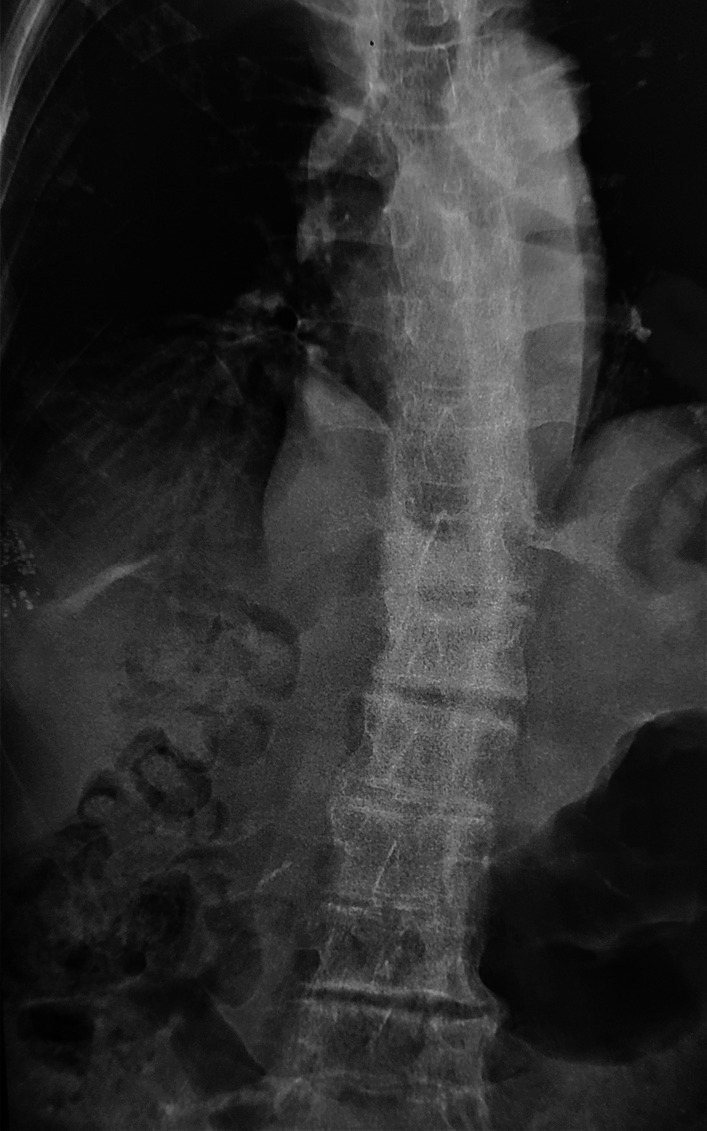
Frontal X-ray of the lumbar spine: syndesmophytes, joint pinching, and diffuse bone demineralization
